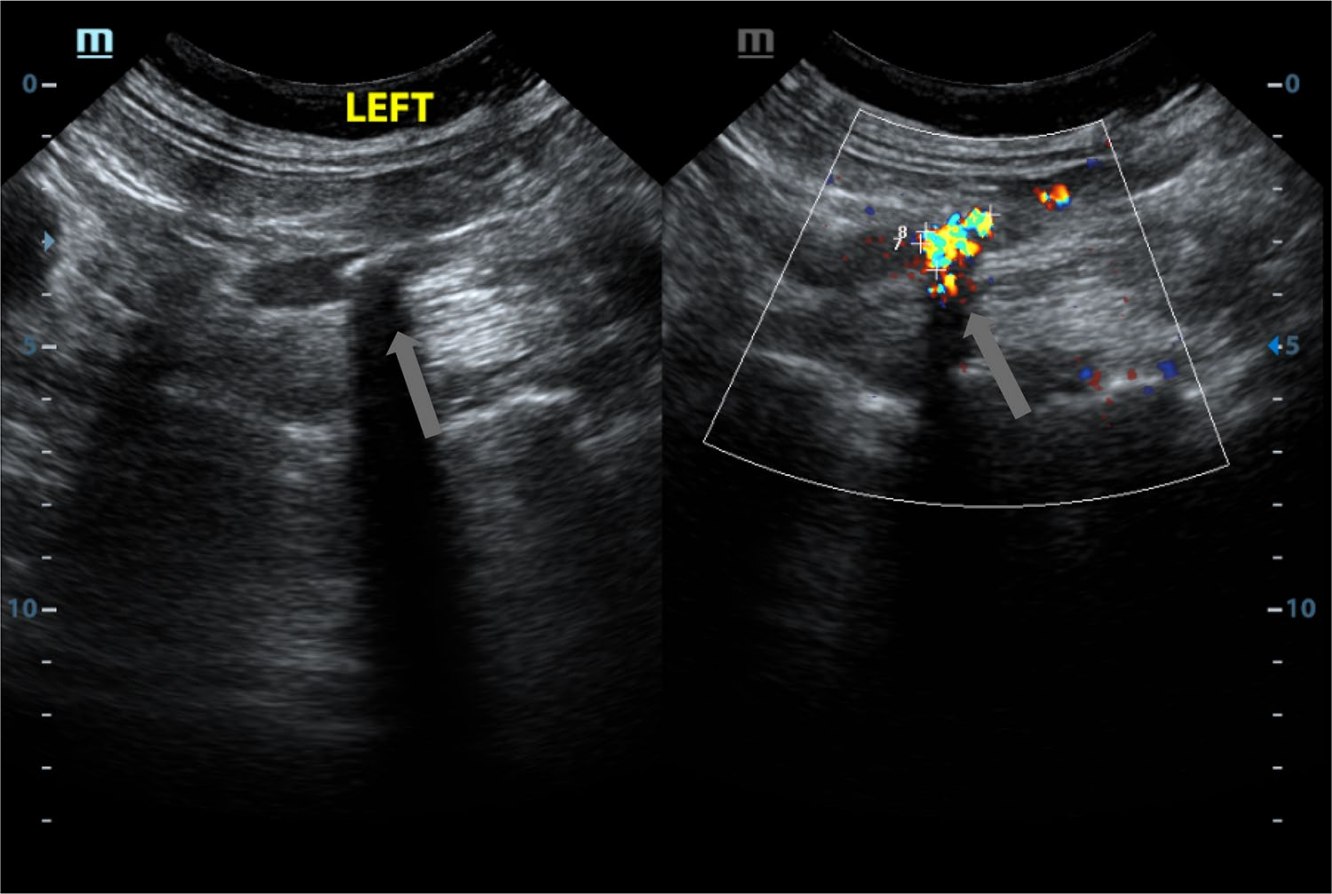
Representative upper ureteral calculus visualized using B-ultrasound (left) and color Doppler twinkling artifacts (right). The B-ultrasound indicated an uneven echo of the stone, and an unclear boundary. The color Doppler twinkling artifacts covered the stone, and the boundaries were clearer
